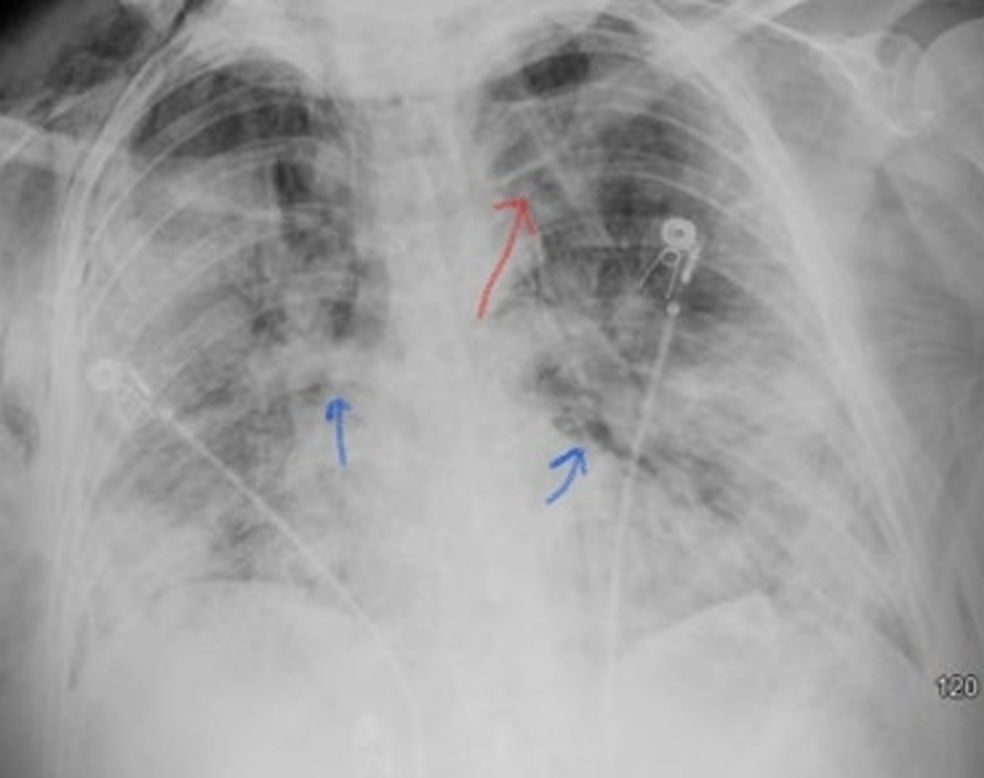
X-ray chest showing marked subcutaneous emphysema, pneumomediastinum (blue arrows), and small left apical pneumothorax (red arrow)
